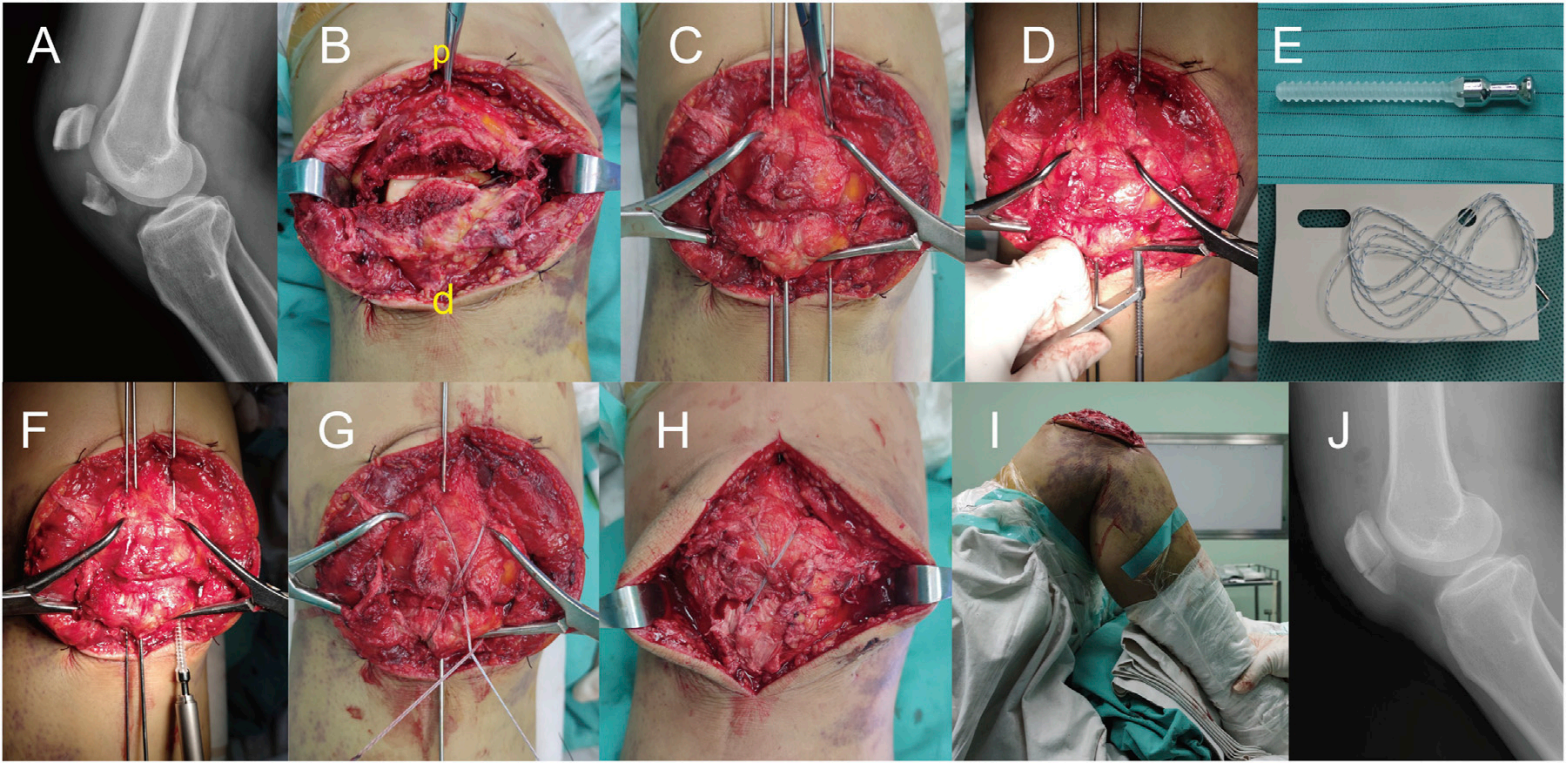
Intraoperative treatment of a transverse patellar fracture with TBSAS. (A) Preoperative X-ray image; (B) Exposed fracture site (p: proximal end of the patella; d: distal end of the patella); (C) Reduction fracture; (D) Expand the tunnels; (E) Absorbable cannulated screw and Ultrabraid high-strength suture; (F) Screw in two 4.5 mm absorbable cannulated screws; (G) Bound and fix the double-strand high-strength suture; (H) Condition after fixation; (I) Passive flexion and extension of the knee joint; (J) Postoperative X-ray image.
